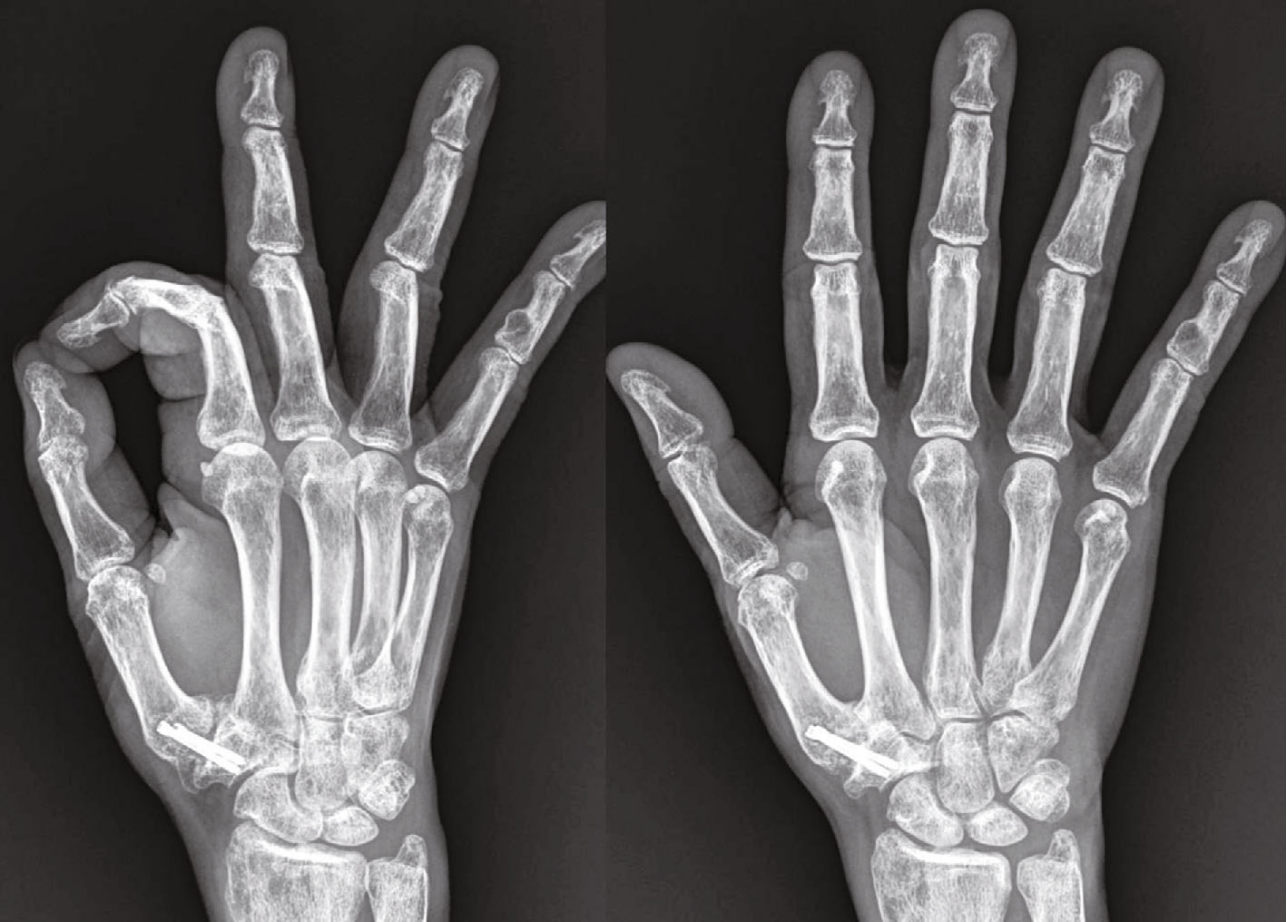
Final radiograph showing the arthrodesis of the trapeziometacarpal joint of the right hand.
